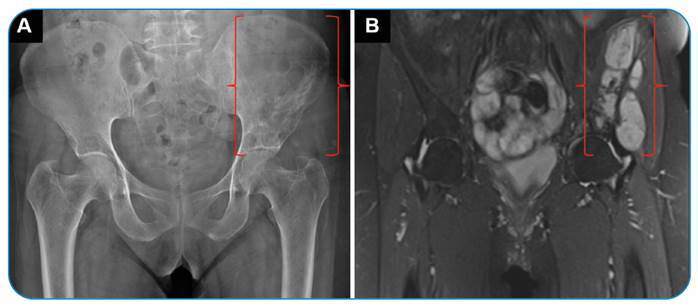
Direct radiography (A) shows a large expansile lytic lesion (in brackets) in the left iliac wing. Magnetic resonance images (B) reveal multicystic iliac lesions (in brackets).
